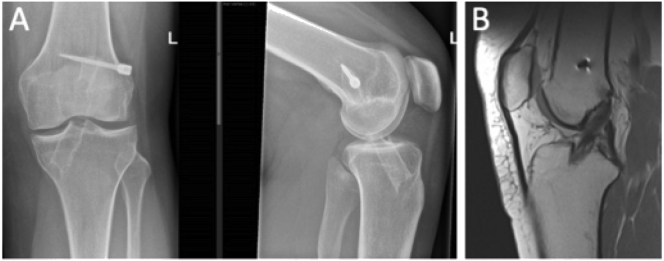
A. Plain X-rays AP and lateral left knee (patient 1). Steep orientation of the graft. B. Sagittal MRI showing intact ACL graft (patient 1).
